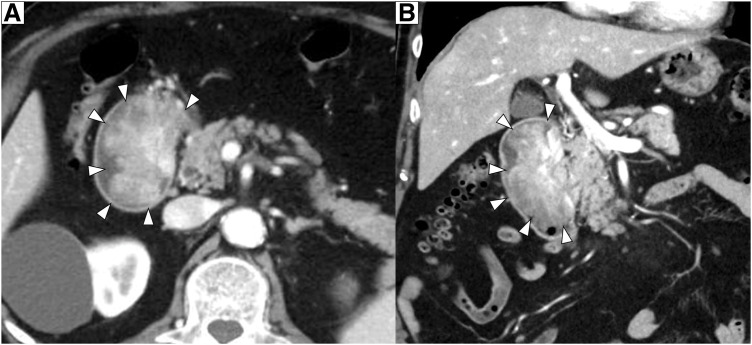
Contrast-enhanced abdominal CT. (A) Axial. (B) Coronal. A 73-mm mass lesion extending from the duodenal bulb to the descending portion of the duodenum was observed (white triangles). A tumor protruding into the duodenal lumen was observed, without clear evidence of extramural invasion.
